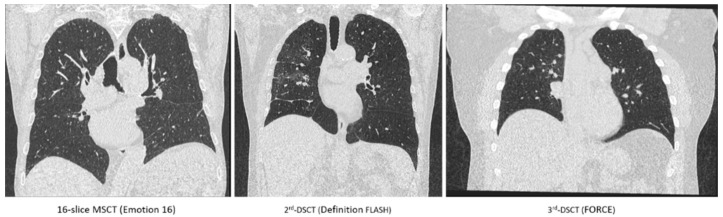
Representative coronal CT sections of the lung reconstructed from data acquired in inspiration for three different patients at the CT systems considered using the scan protocols summarized in Table 1 (identical windowing of Hounsfield values).
